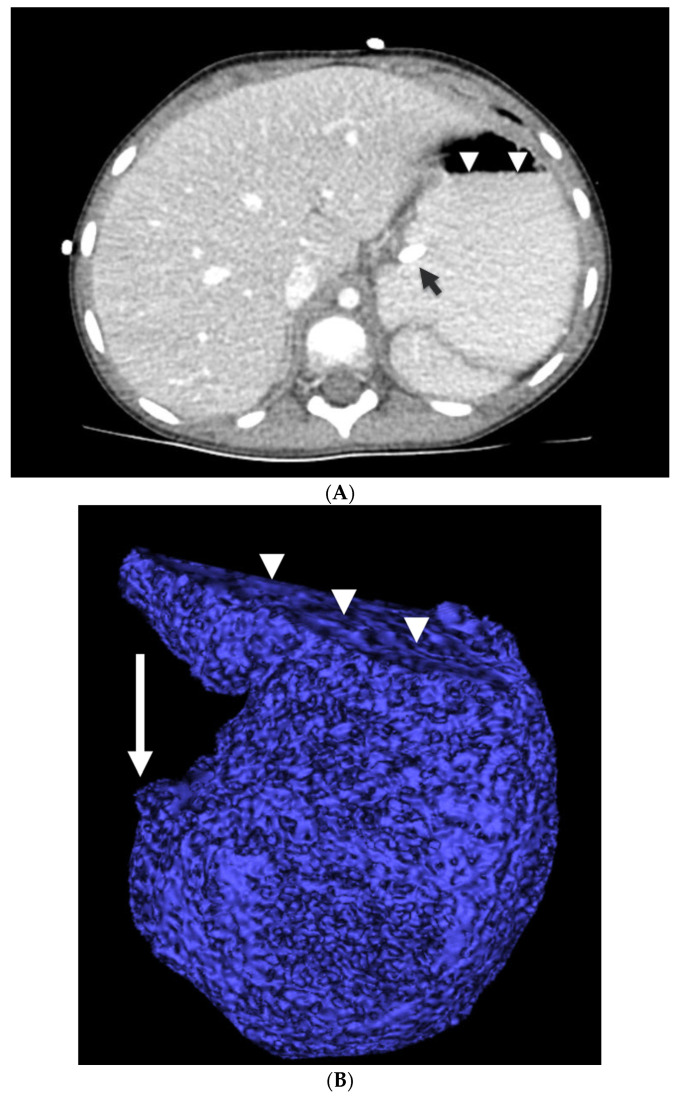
(A) Axial 3 mm slice from the CT shows the outline of the dependent fluid filled in the stomach by the arrowheads. The short black arrow points to the enteric tube. Contrast fills the fundus of the stomach and is in contact with the gastroesophageal junction. (B) Three-dimensional surface-rendered volume of the stomach contents. Arrowheads indicate the air–fluid interface. The arrow shows the location of the pylorus and the greatly distended antrum proximal to the pylorus. The total volume measures 132 mL. The average density is 168 Hounsfield units, indicating contrast mixed with secretions.
